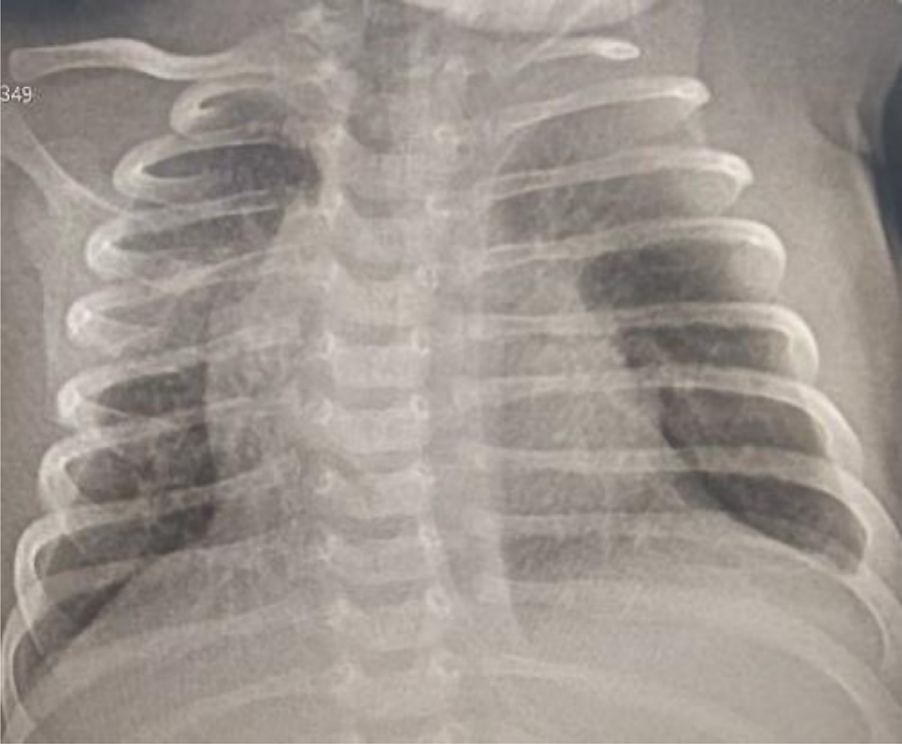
Postoperative frontal chest radiograph shows expansion of the previously collapsed lower lobes and the right upper lobe, and resolution of the mediastinal shift.
